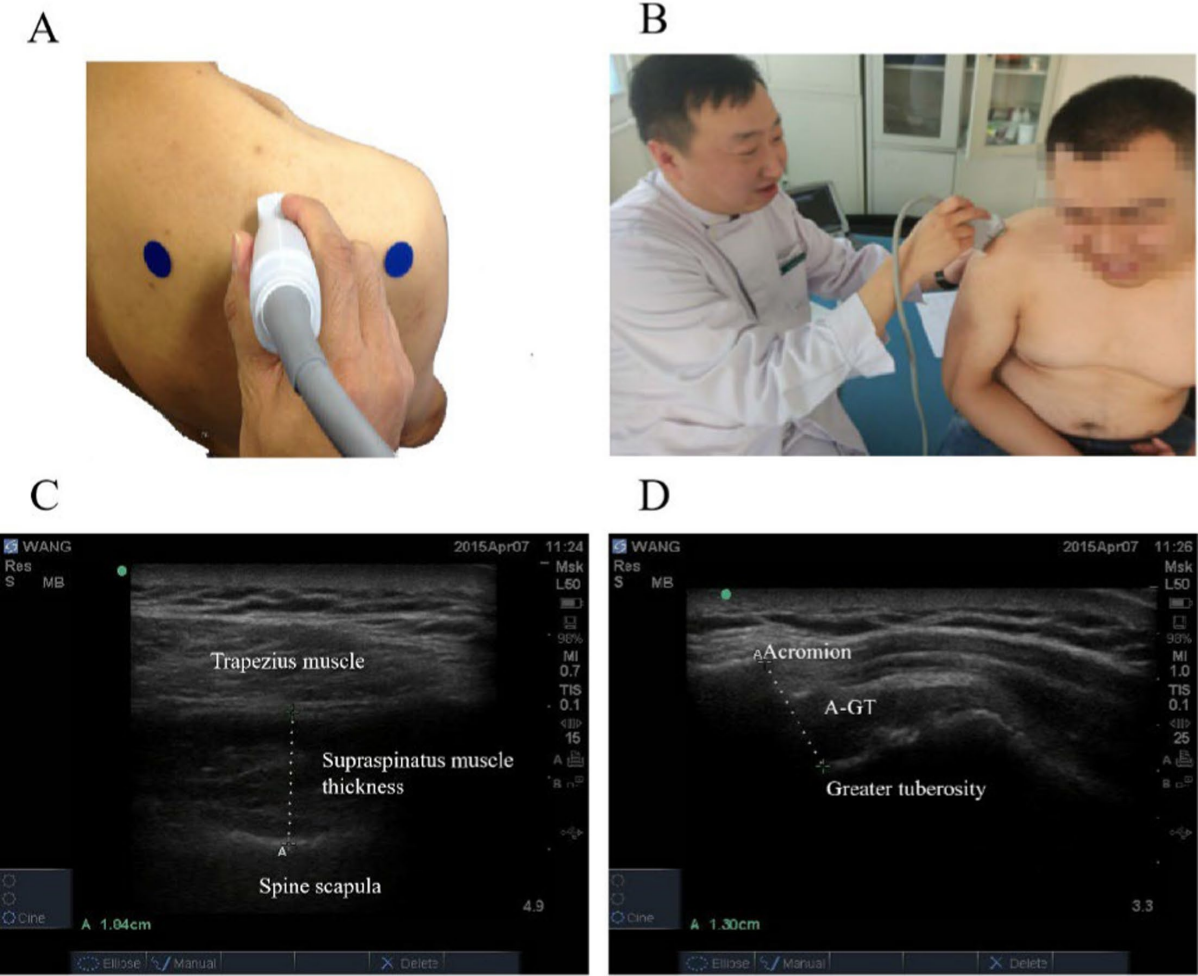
The supraspinatus muscle thickness, AGT were measured by ultrasound imaging. (A) The ultrasound probe was placed vertically at the midpoint of the scapula and moved parallel to the thickest part of the supraspinatus muscle. (B) The ultrasound probe was placed vertically between the acromion and the upper part of the greater tuberosity of the humerus. (C) Supraspinatus muscle thickness. (D) Acromion-greater tuberosity distance.
